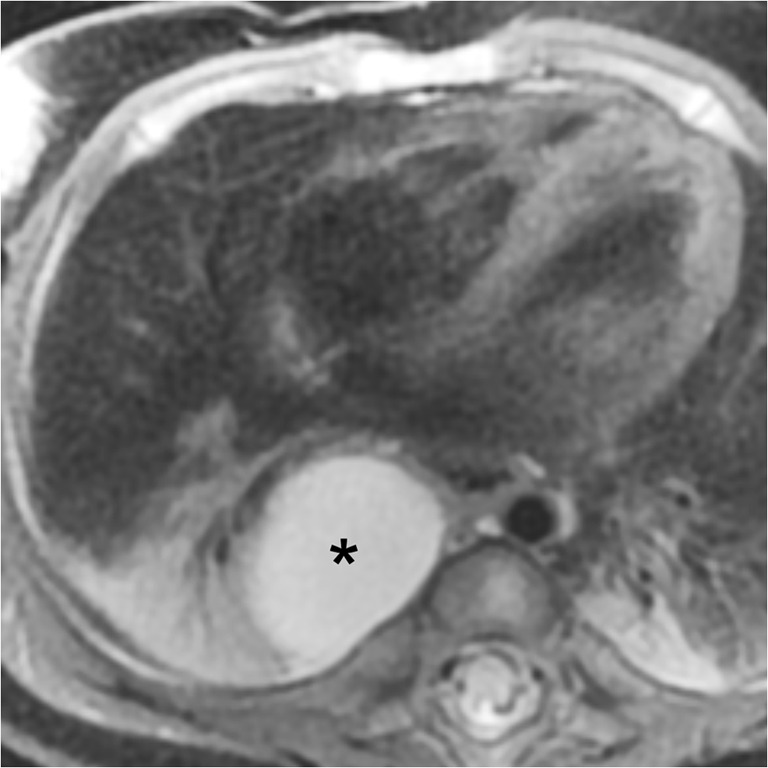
Oesophageal duplication cyst in a 3-month-old girl with foetal ultrasound diagnosis of cystic congenital pulmonary airway malformation. Axial fat-saturated T2-weighted image shows broad connection of the unilocular fluid-filled cyst (*) with the oesophageal wall
